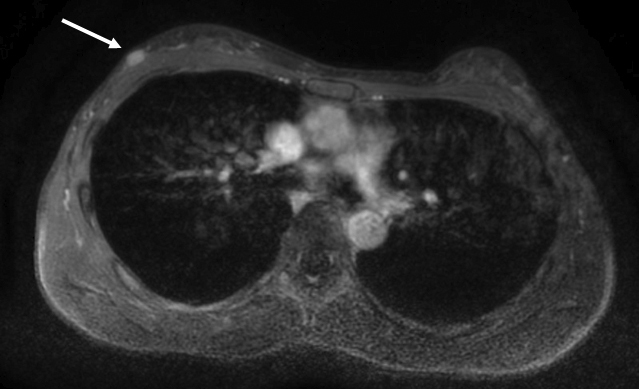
Magnetic resonance imaging (MRI) revealed an 8-mm oval mass with circumscribed margins in the upper outer quadrant of the left breast (arrow). Dynamic contrast-enhanced MRI demonstrated homogeneous enhancement with a fast washout pattern.
